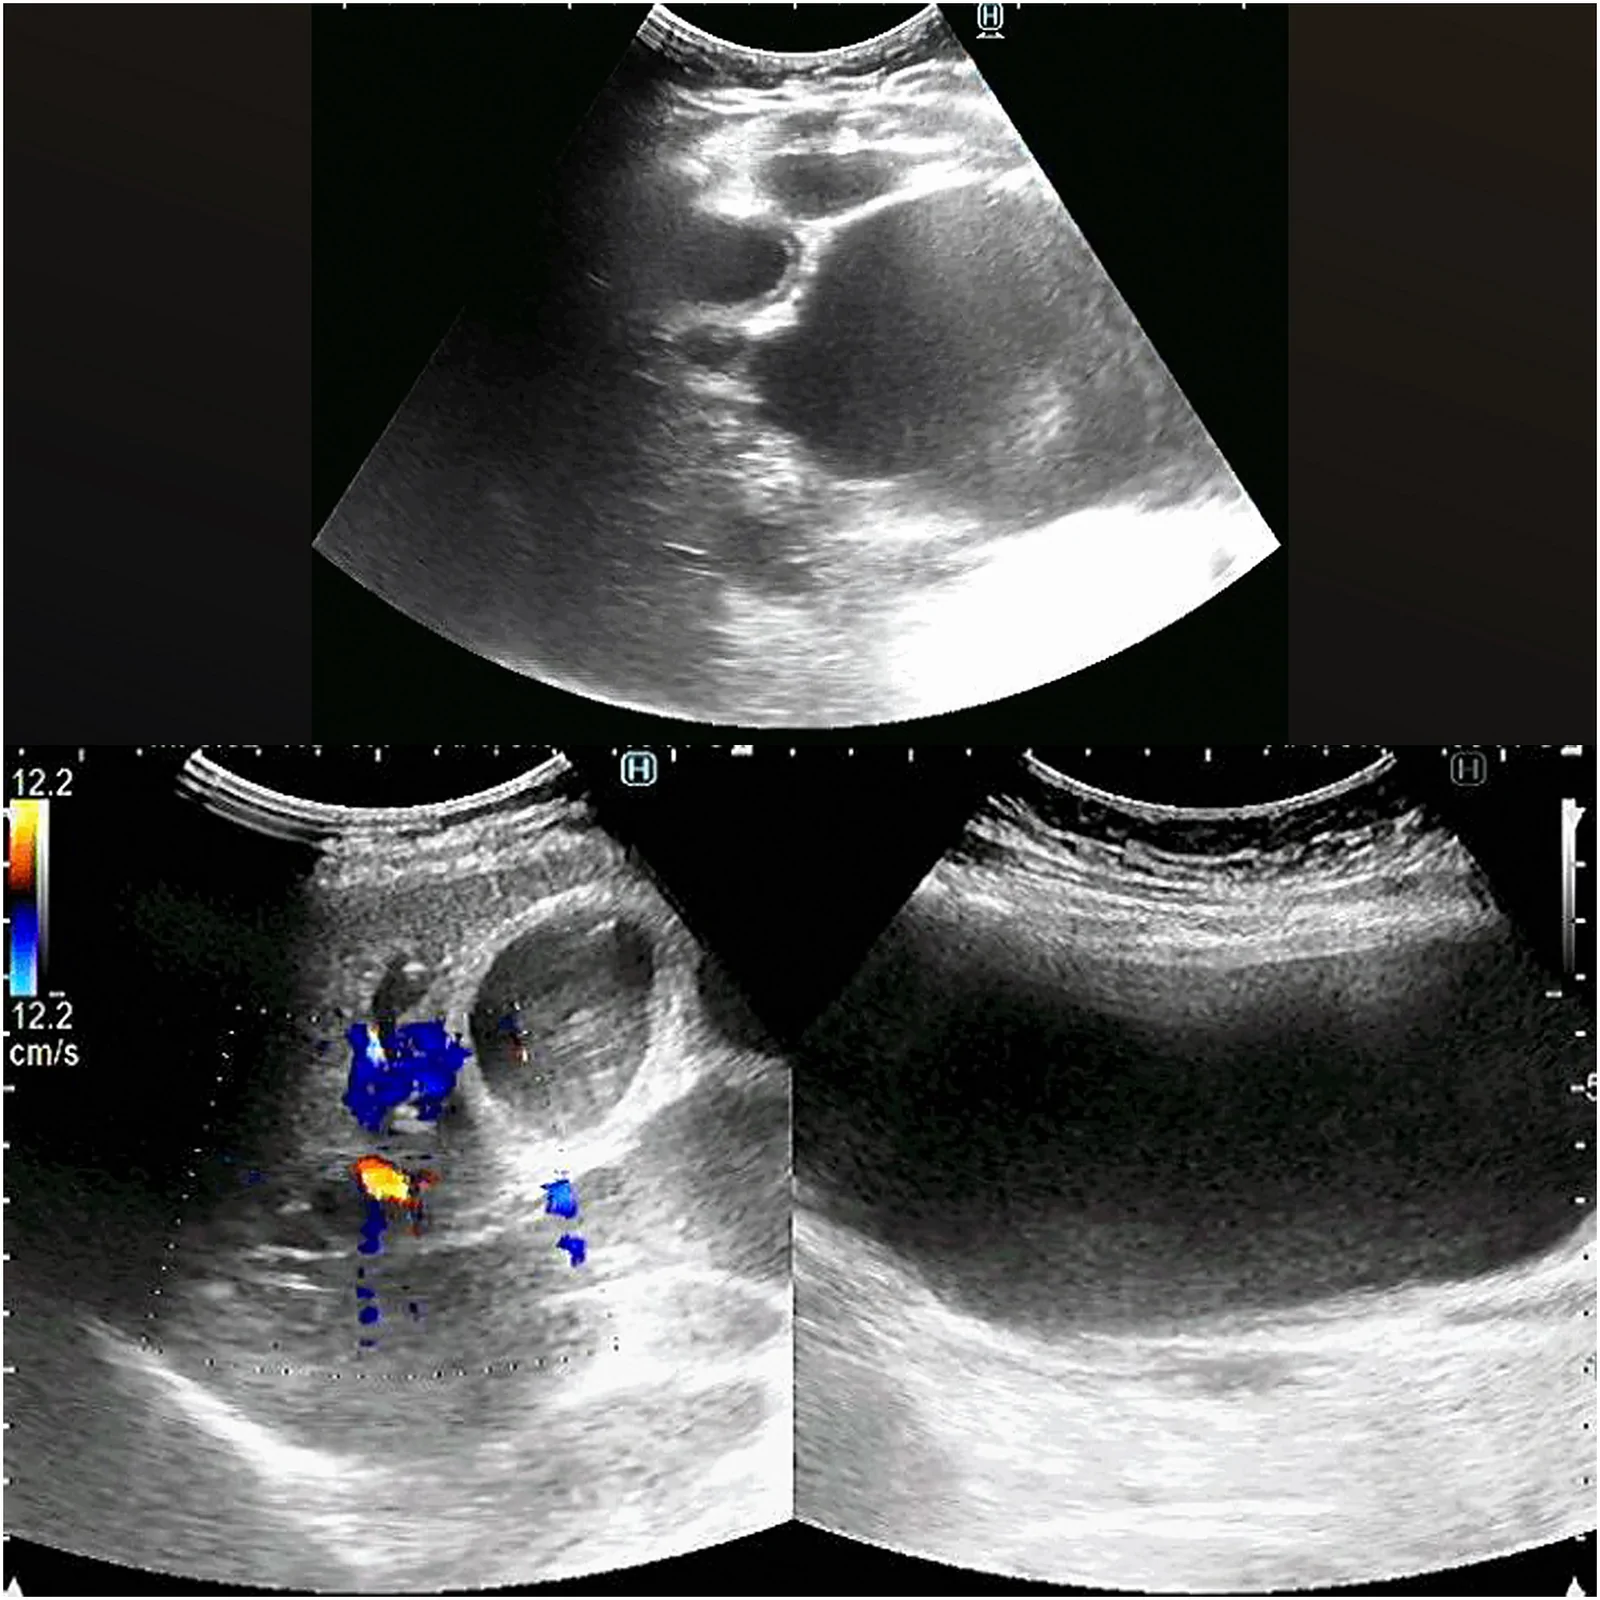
Abdominal ultrasonography showing a giant cystic extrahepatic bile duct in the subhepatic region, associated intrahepatic biliary dilatation, and gallbladder distension.
